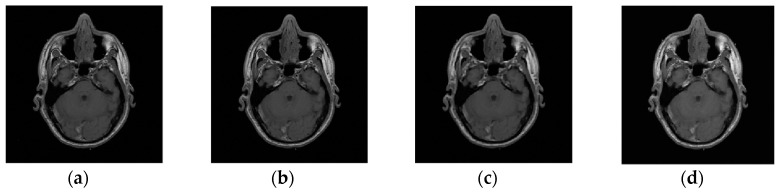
Reconstruction results for the magnetic resonance imaging (MRI) image, considering 45% the missing information; the results are obtained using the (a) gradient; (b) radial-Fourier; (c) TV-min-DCT; and (d) Douglas–Rachford algorithms.
